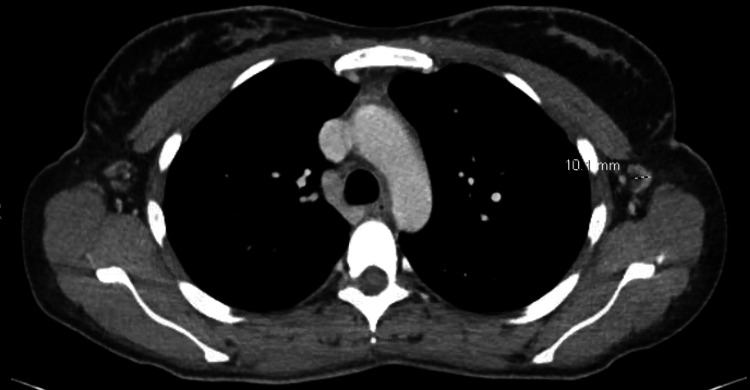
CT of the chest demonstrating left axillary lymphadenopathy.
